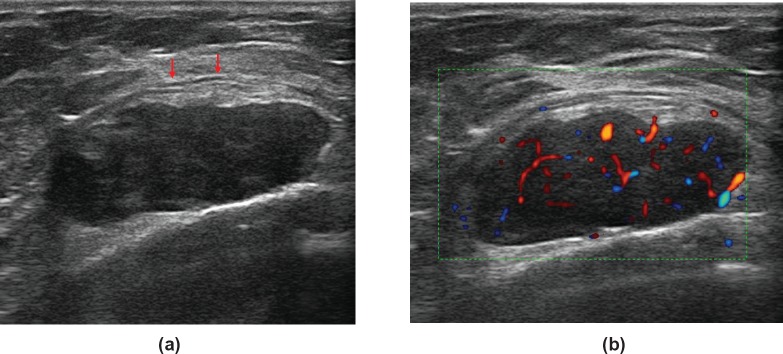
A 56-year-old woman with neurofibroma of the right sulcus: (a) US reveals a solid mass, oval-shaped, with circumscribed margins; the mass abuts the chest wall without signs of infiltrations (arrows). (b) Colour-Doppler shows high vascularity.
